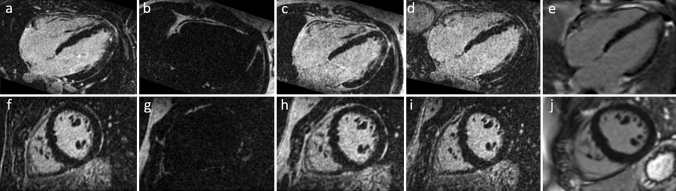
3D Dixon LGE MR imaging of a healthy patient at 1.5 T showing water-only (a, f), fat-only (b, g), in-phase (c, h), opposed phase (d, i) acquisitions and the 2D magnitude images (e, j) in 4-chamber (top row) and short axis views (bottom row)
